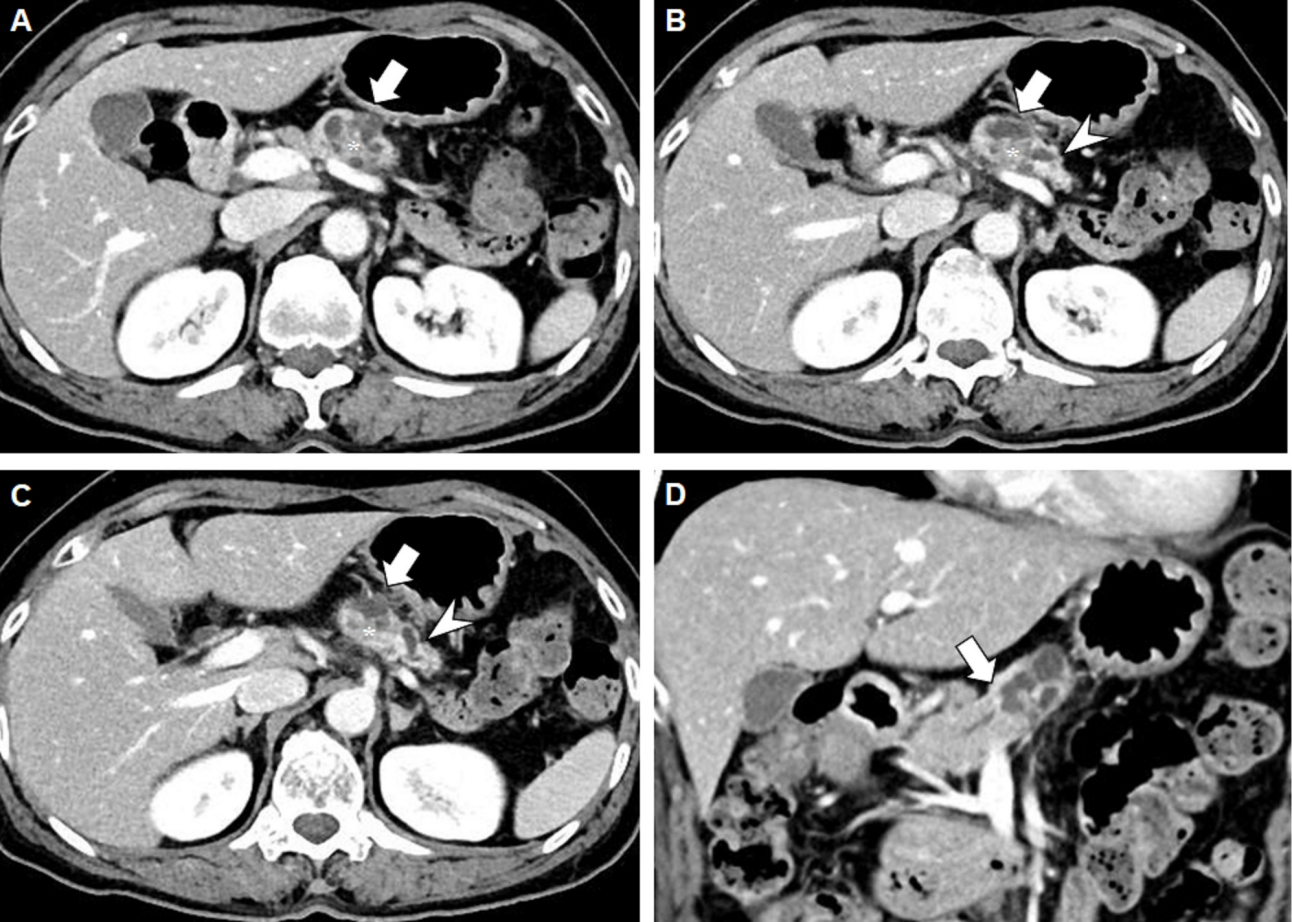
Representative example of CT findings of HG PanIN in a 65-year-old woman with malignant IPMN. A-C. The portal phase axial CT image obtained preoperatively shows a 31-mm pleomorphic cystic mass in the pancreas body (arrow). It contains an enhancing mural nodule 21 mm in diameter (asterisk). Note that the upstream MPD is dilated up to 6 mm in diameter with parenchymal atrophy (B and C, arrowheads). D. The portal phase coronal CT image shows an abrupt diameter change in the MPD (arrow) due to the lesion.
